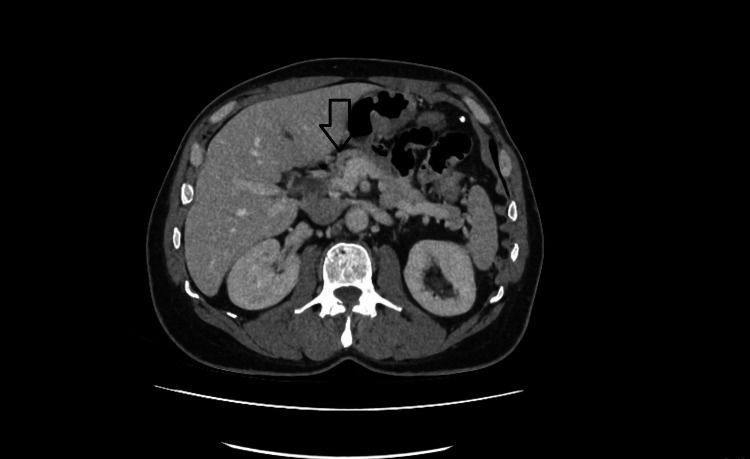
CT scan of the pancreas showing a 1.25 cm enhancing lesion in the proximal body of the pancreas
